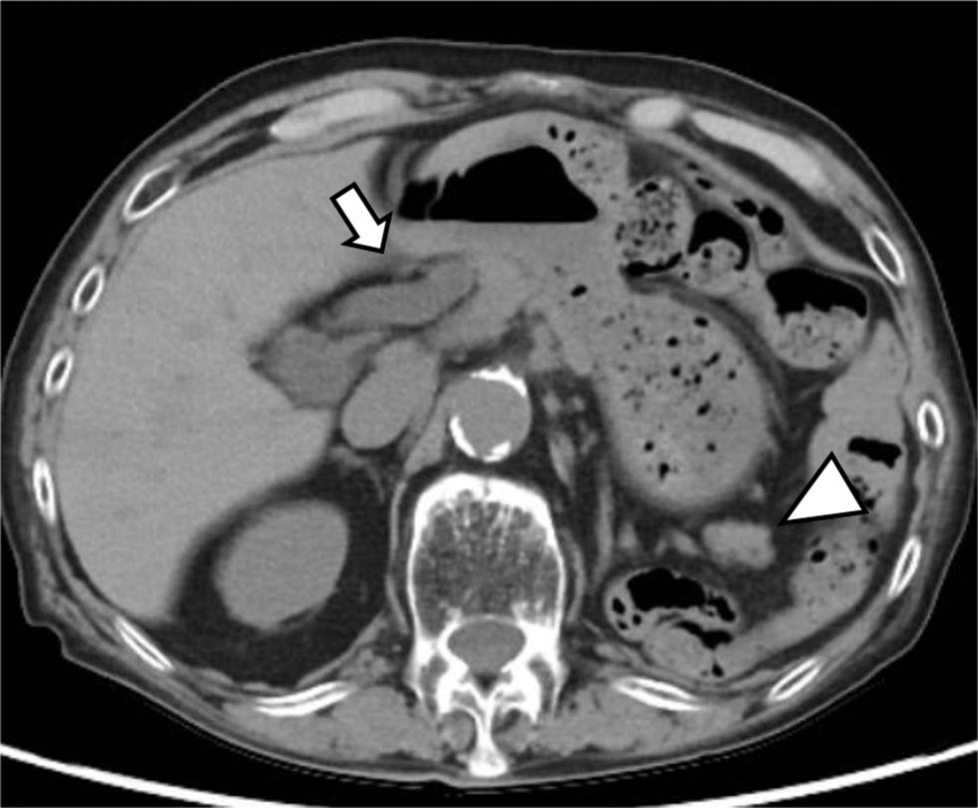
Non-contrast CT after PSL therapy. Non-contrast CT after PSL therapy shows the improvement in the bile duct wall thickening (arrow). The pancreas tail enlargement is also diminished (arrow head)
